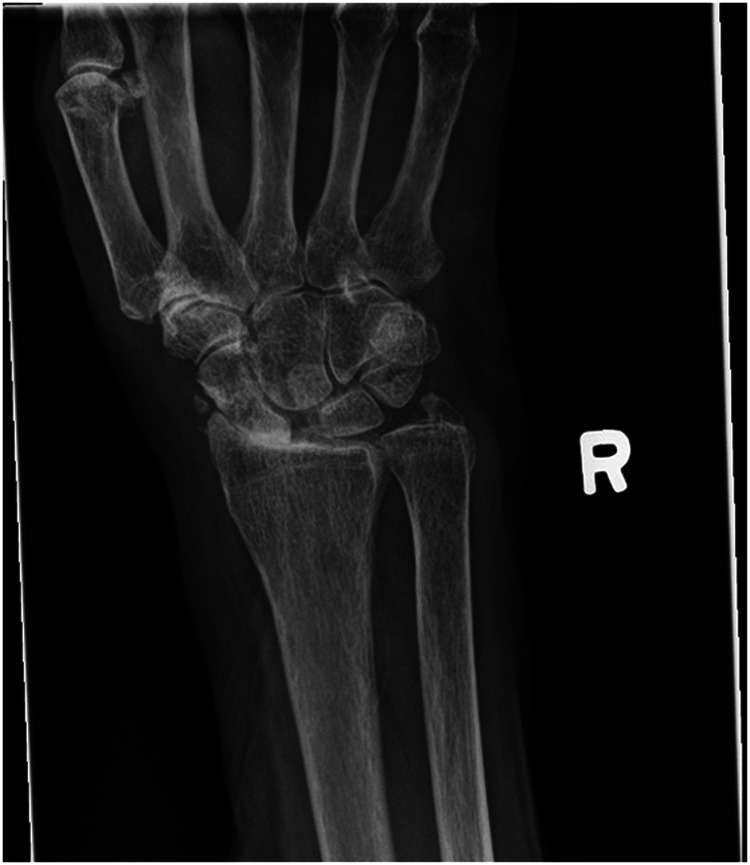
Preoperative right hand plain film posterior to anterior view x-ray.
